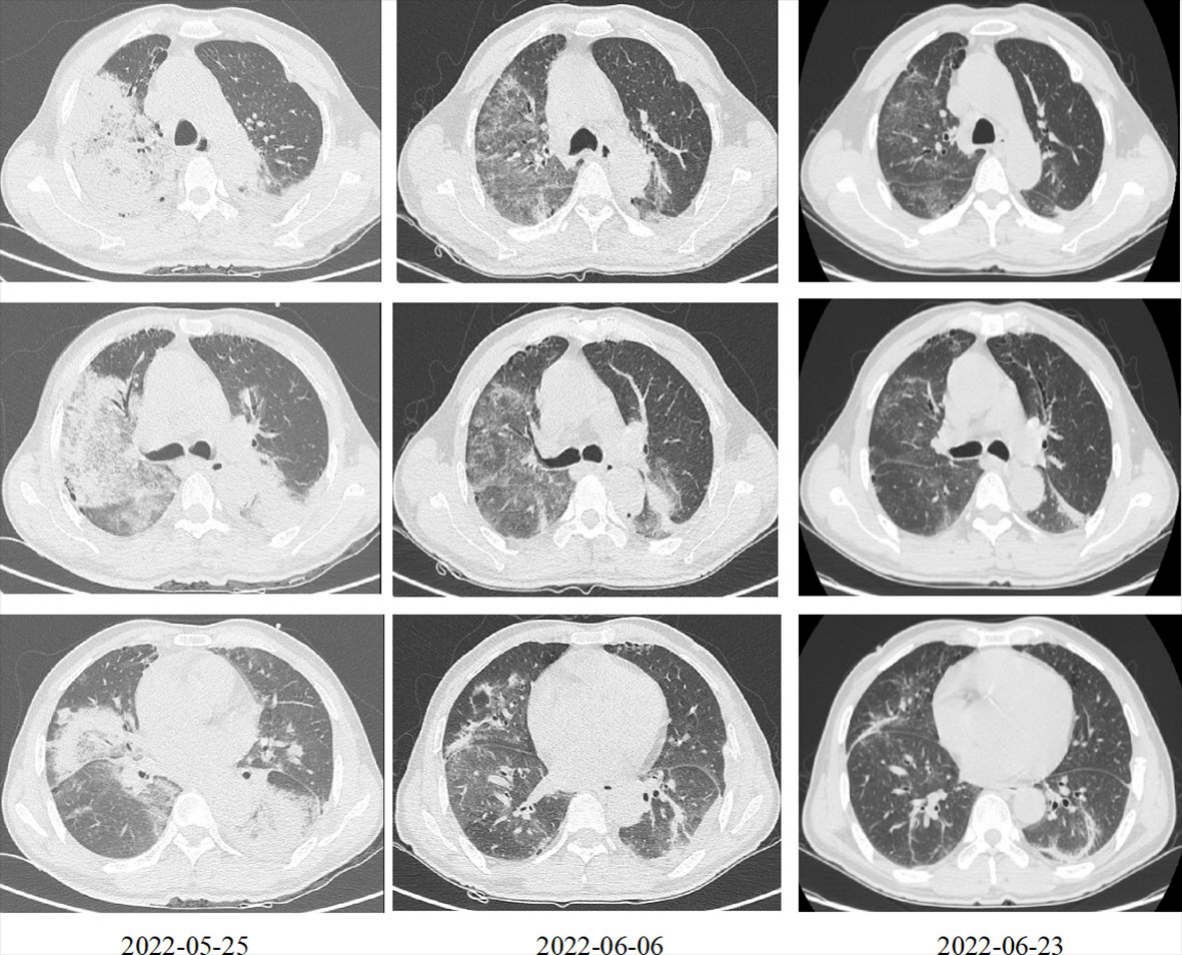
Chest computed tomography at different time points after treatment. March 25, 2022: Bilateral multiple exudative foci in the lungs, large consolidation opacities, and a small amount of bilateral pleural effusion. June 6, 2022: The exudative foci of both lungs were less absorbed than before. June 23, 2022: The exudative foci in both lungs were further absorbed as compared to before.
